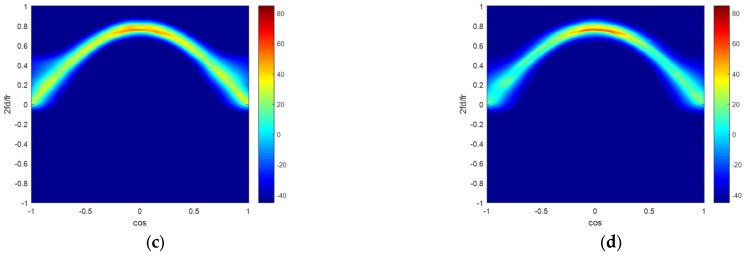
Clutter Angle-Doppler capon spectrums estimated by different methods in the non-ideal condition with ICM: (a) the ideal CNCM; (b) SMI; (c) KASPICE-STAP; (d) the proposed method.
